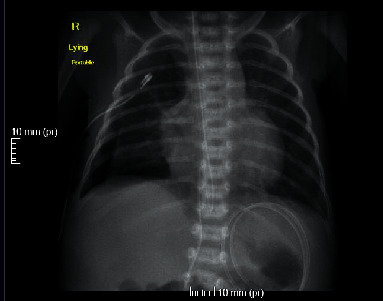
Chest X-ray after insertion of right-sided intercostal tube and octreotide infusion.
